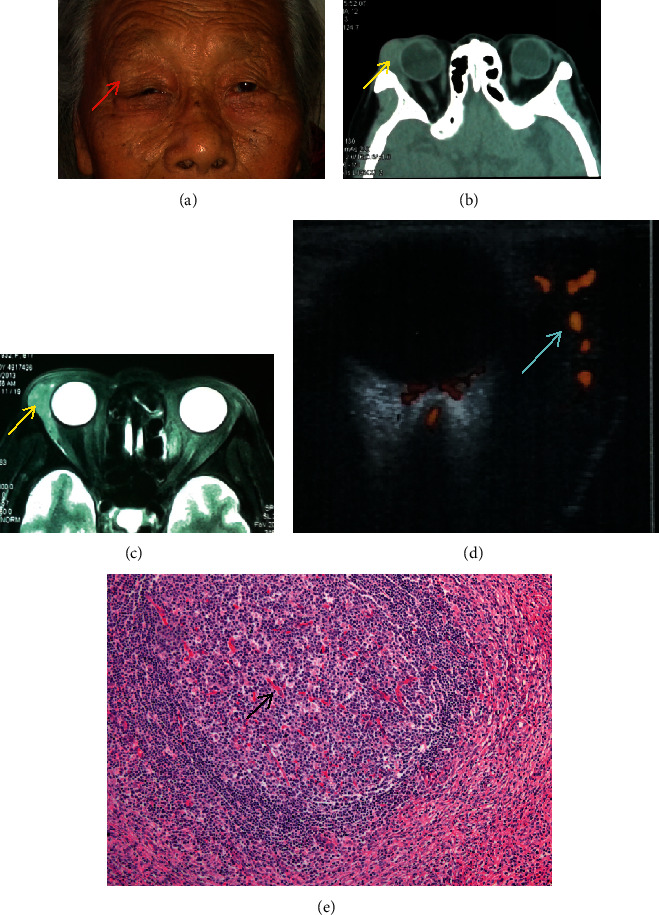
(a), (b), (c). Preoperative appearance of the right eye. CT and MRI showed a swollen mass in the lacrimal gland area (red and yellow arrows). (d) Color ultrasonography showed a mass in the lacrimal gland area of the right eye with abundant blood flow (blue arrow). (e) Small blood vessels were observed invading the germinal center (black arrow).
